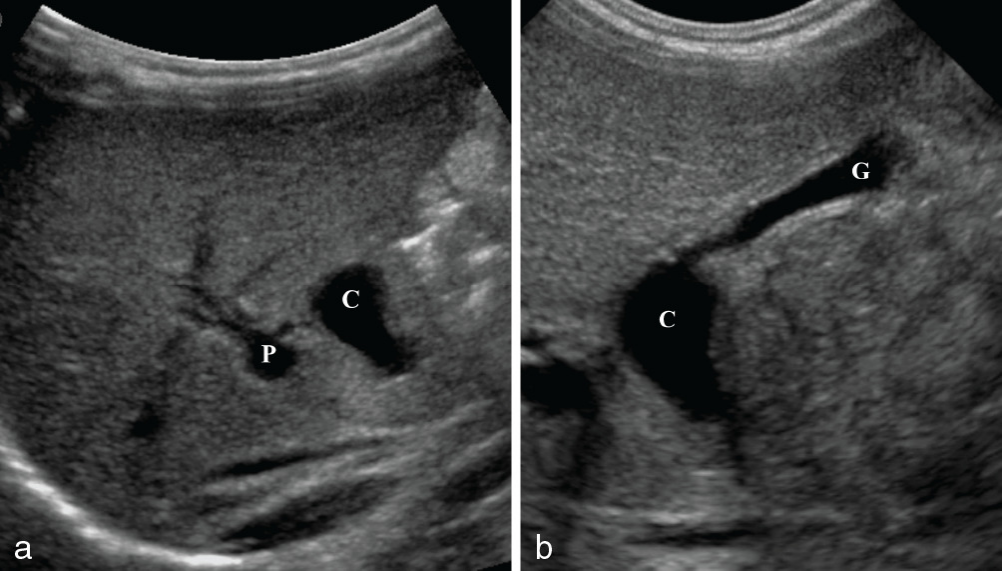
Ultrasound of the abdomen of case 1 showing (a) an anechoic extrahepatic cystic structure (C) adjacent to the main portal vein (P). (b) This cyst (C) was connected to a small and elongated gallbladder (G).
